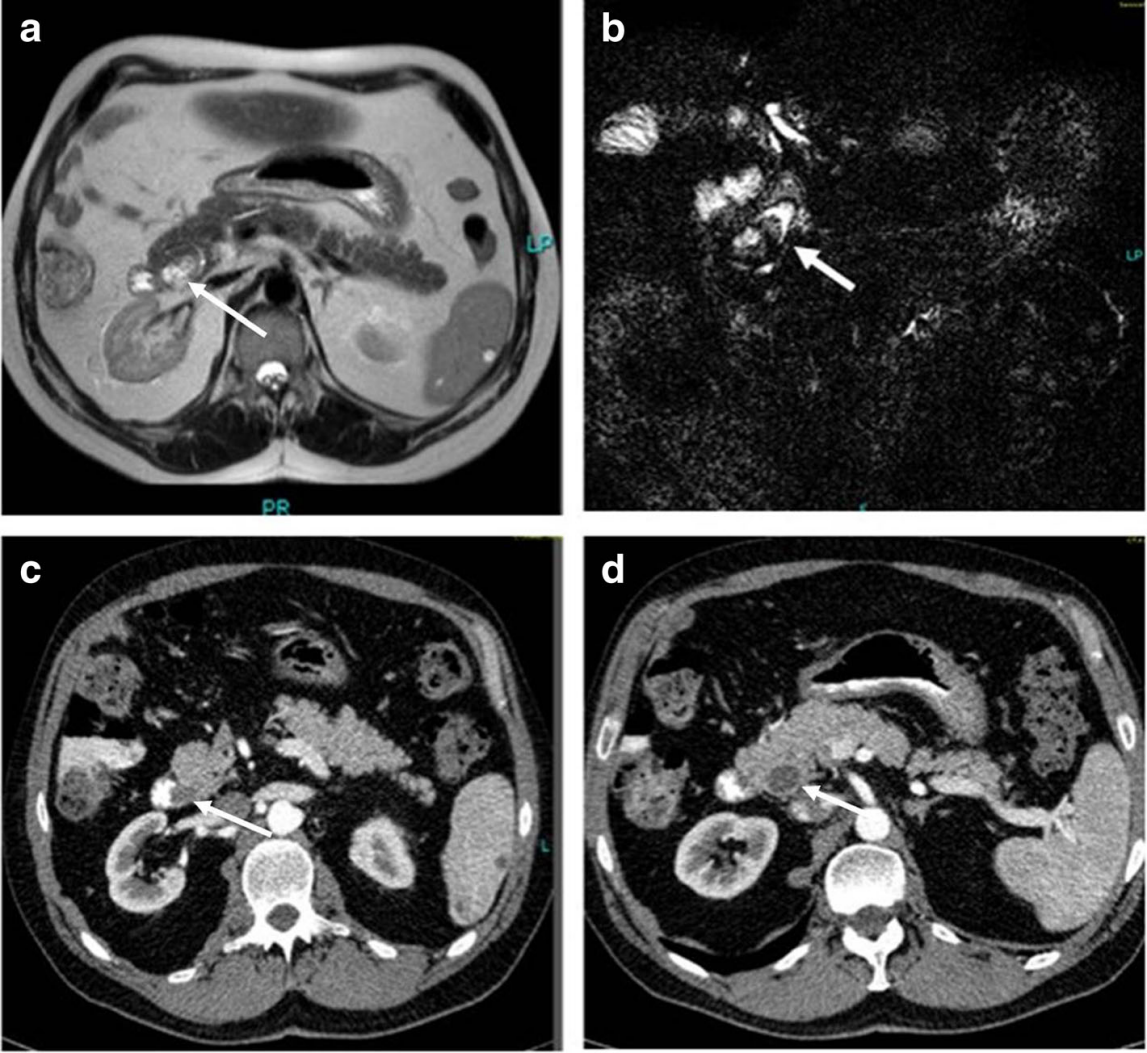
a Magnetic resonance imaging scan showing a contrast-enhanced mass in the pancreatic head (*arrow*). b Magnetic resonance cholangiopancreatography showing signal loss of the bile duct (shown by *white arrow*). c Computed tomography scan showing a mass near the papilla of Vater (*arrow*). d Computed tomography scan showing the dilation of the residual main bile duct (*arrow*)
